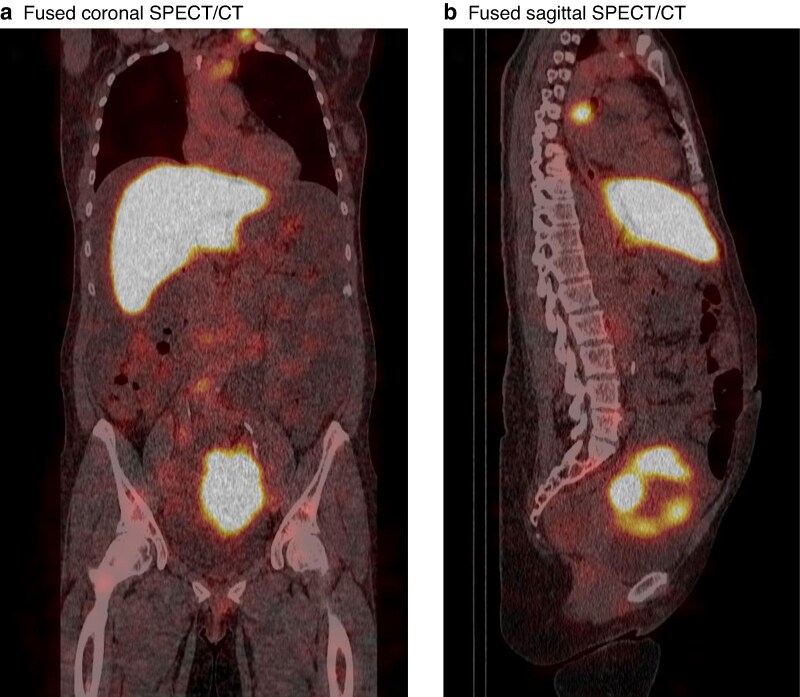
Multimodal imaging of previously undetected lymph node metastases (patient 7) a Retroclavicular node, b para-oesophageal node. SPECT, single-photon emission computed tomography; CT, computed tomography.
